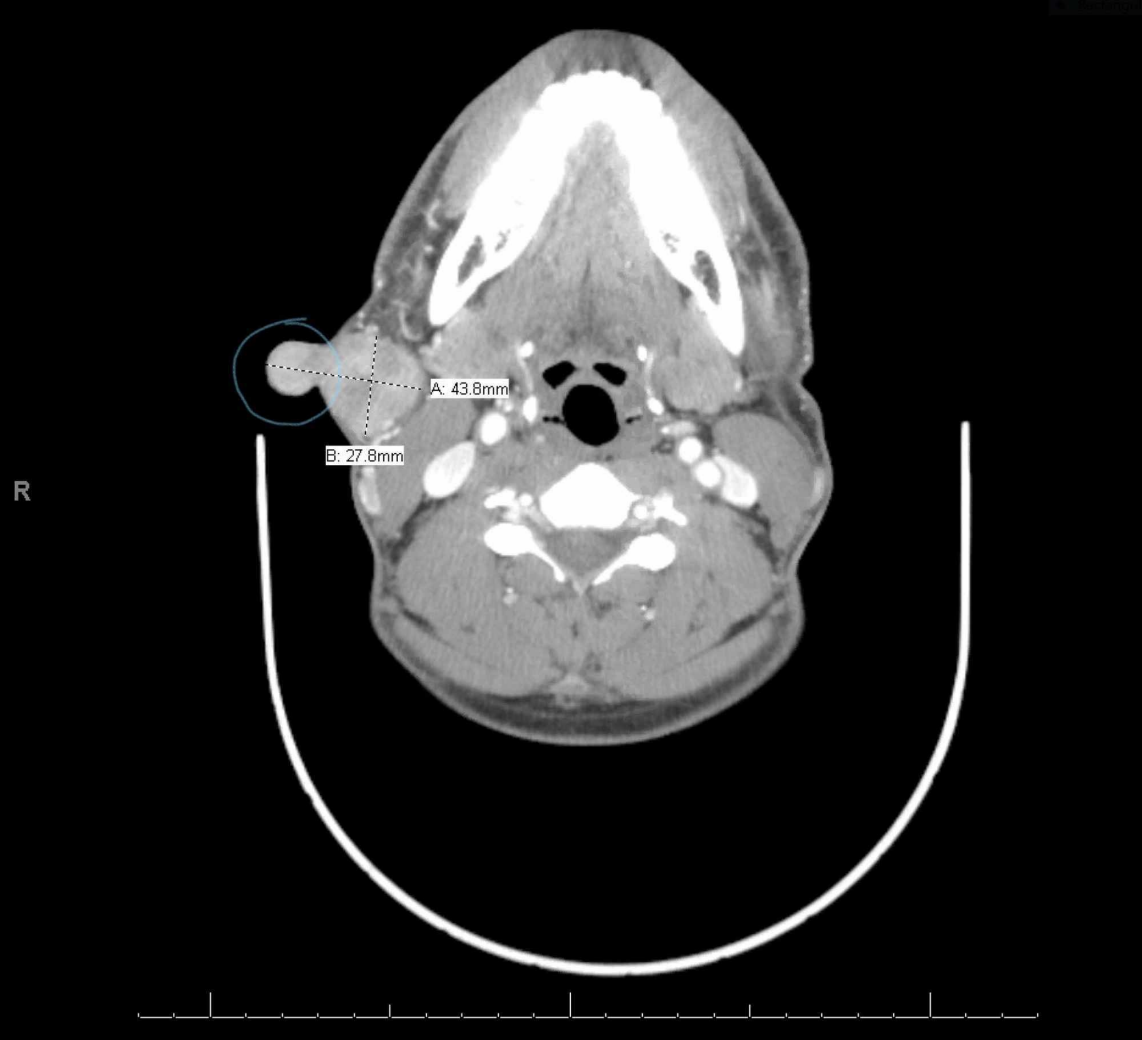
CT neck with contrast showing an avidly and heterogeneously enhancing right neck mass measuring 4.38 cm X 2.78 cm X 3 cm, at the level of the hyoid bone, superficial to the platysma with no overt invasion through the platysma. The blue circle drawn on the nodule is the externally (clinically) visible part measuring 1 X 1 cm (at the time of the computed tomography (CT) scan).
